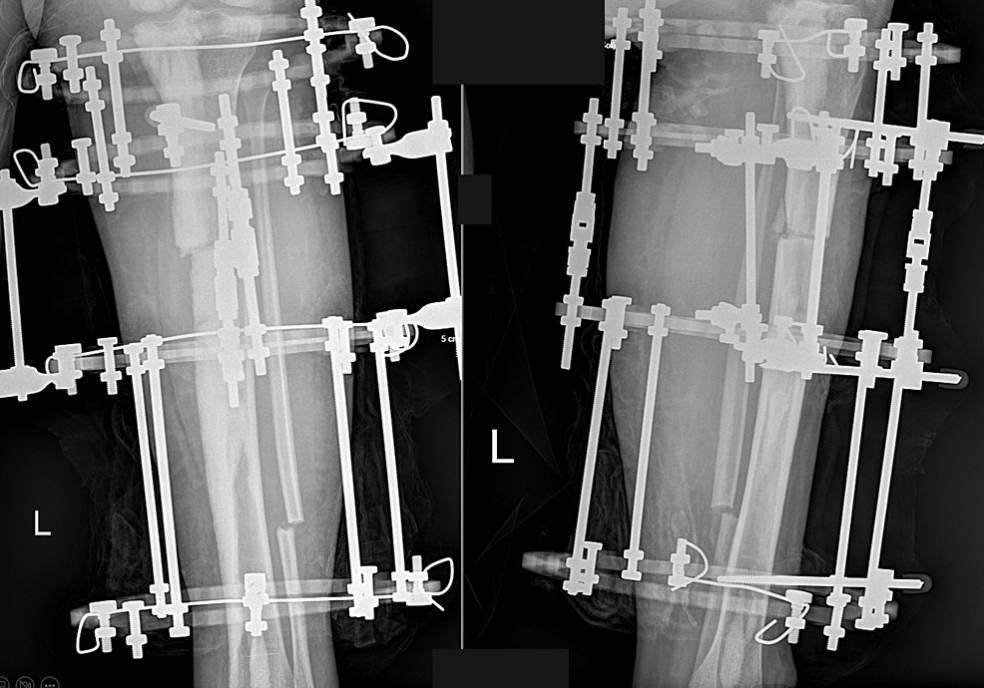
Left tibia anterior-posterior and lateral plain radiograph after the surgical intervention. As seen in the plain radiographs, deformity correction is obtained via the circular frame of Ilizarov.
